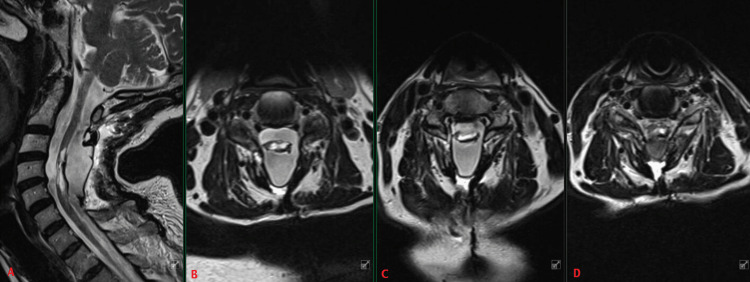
Postoperative MRI of the cervical spine with mid-sagittal (A) and selected axial T2-weighted images at C3/4 (B), C4/5 (C) and C5/6 (D) demonstrating a more ventral position of the cord compared to the preoperative imaging studies, and restoration of space and CSF fluid volume within the dorsal cistern. There is increased space between the spinal cord and the reconstructed dura, which has been facilitated by the “billowing duraplasty.” Note the presence of the retained, adherent dural placode on the dorsal aspect of the spinal cord, represented by the region of low signal on the T2-weighted images, best seen on the mid-sagittal slice.
